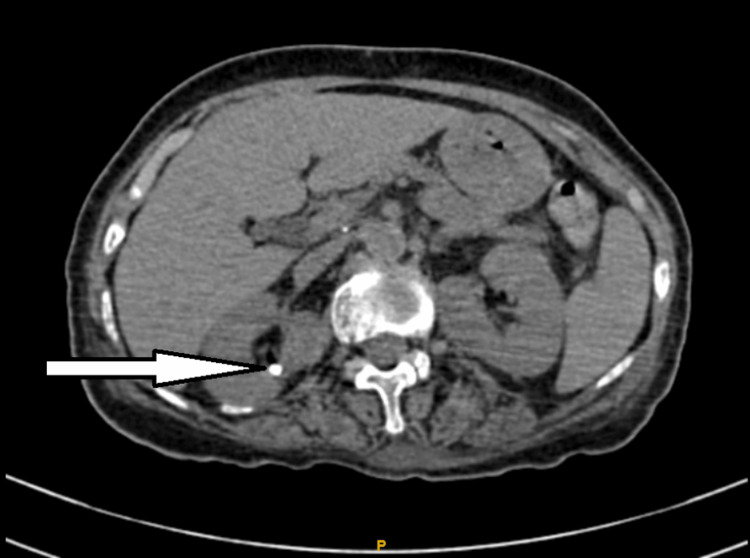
CT scan showing degenerative changes in the spine and hyperdense calculi in the right kidney CT, computed tomography
